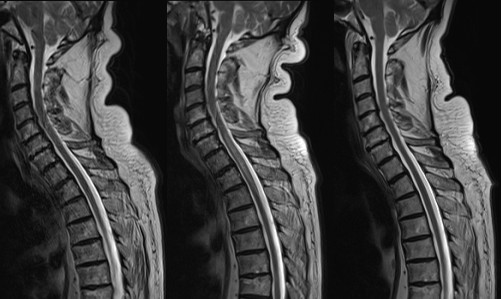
Chronological MRI images of thoracic spine - November 2008/December 2008/May 2009. In figure 2 the thoracic spine is most clearly affected in the second image (December 2008). The images show increased signal within the substance of the spinal cord during episodes of active vasculitis, with some subtle expansion of the cord.
